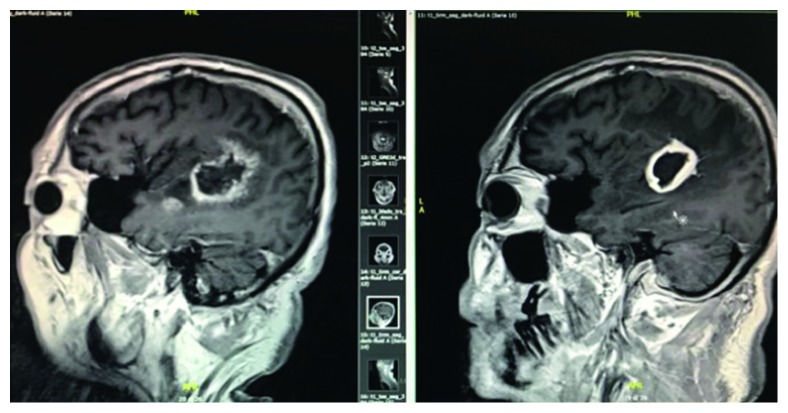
MR image showing the evolution of the ring-enhancing lesion in the left fronto-temporoparietal lobe in a brain abscess with significant perilesional edema.
